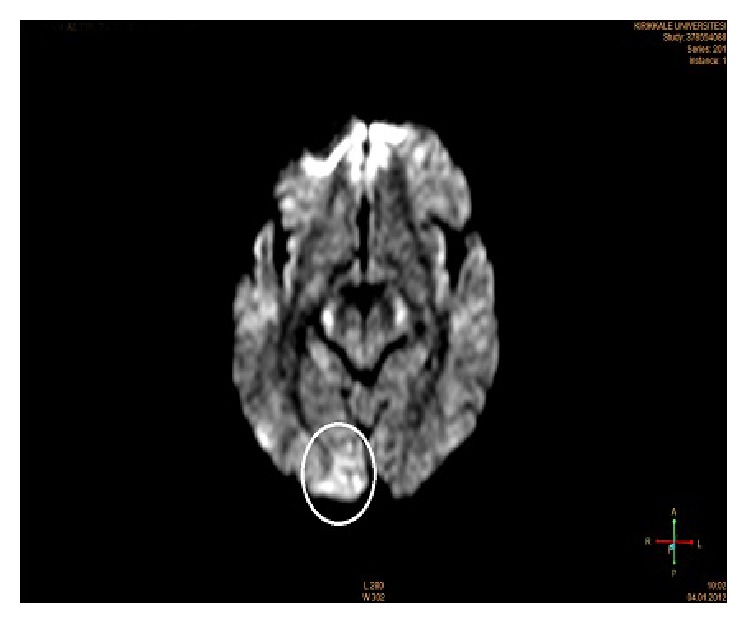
Axial diffusion-weighted echo-planar MR image (apparent diffusion coefficient shows an area of restricted diffusion in right occipital lobe).
